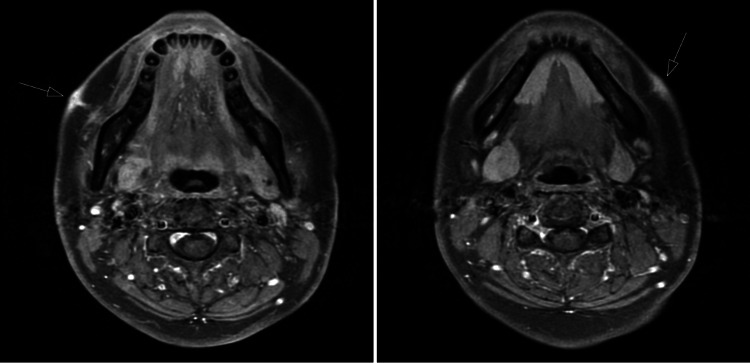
MRI findings at a three-month follow-up Notable improvement of the sinus tracts in the right buccal space and left perimandibular soft tissues following empiric antibiotic therapy, which, in combination with the patient’s preference to avoid surgery, significantly influenced the decision to continue antibiotic therapy for 12 months with appropriate clinical and radiographic monitoring.
